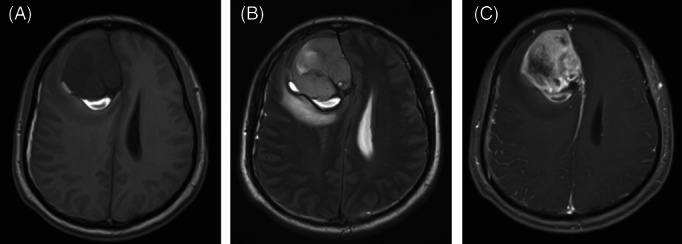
Magnetic resonance imaging showed a mass located in the right frontal region with heterogeneous isointense T2‐weighted (A) and hypointense T1‐weighted (B) signal. After gadolinium administration, the mass showed heterogeneous enhancement (C).
